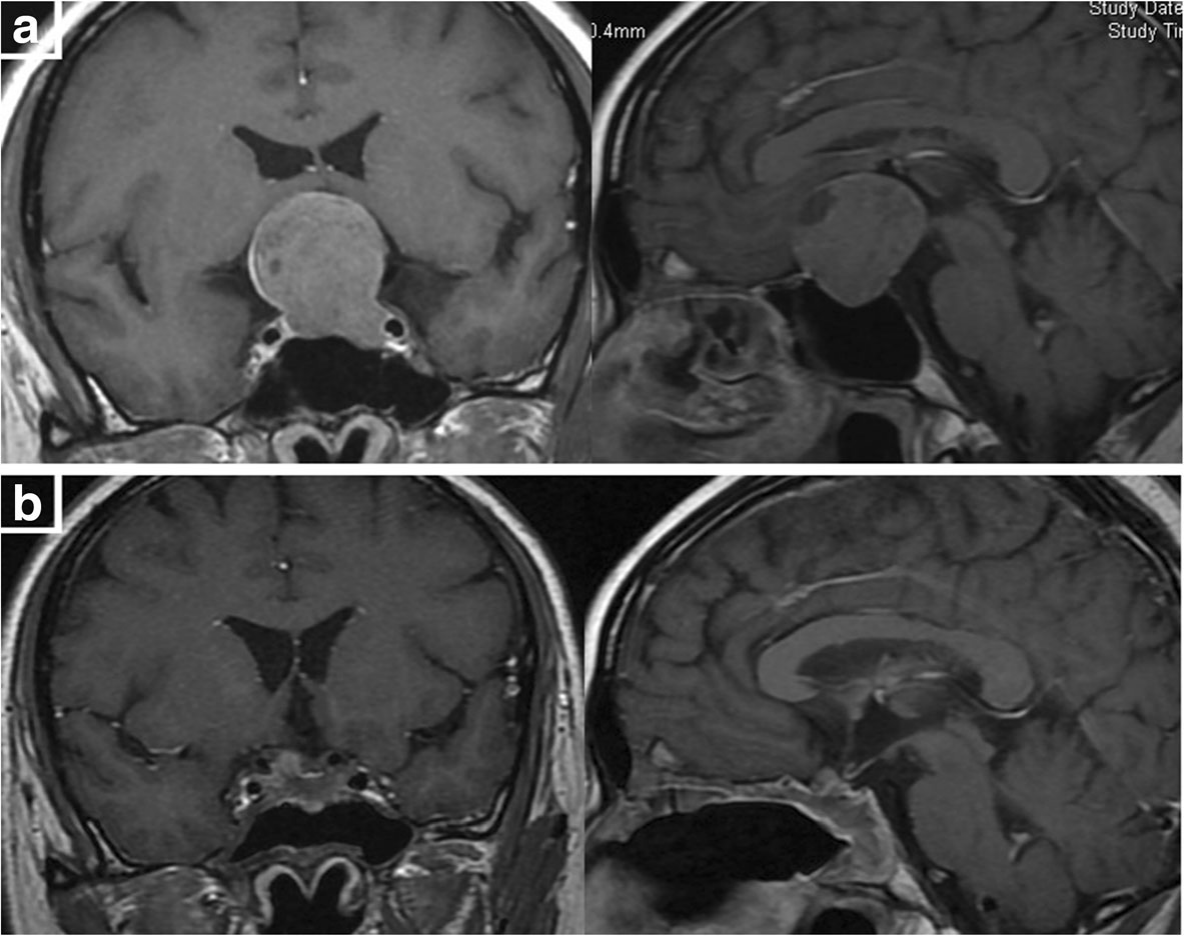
a preoperative MRI shows giant pituitary adenoma, growing expansively. b postoperative MRI shows tumor was totally removed
